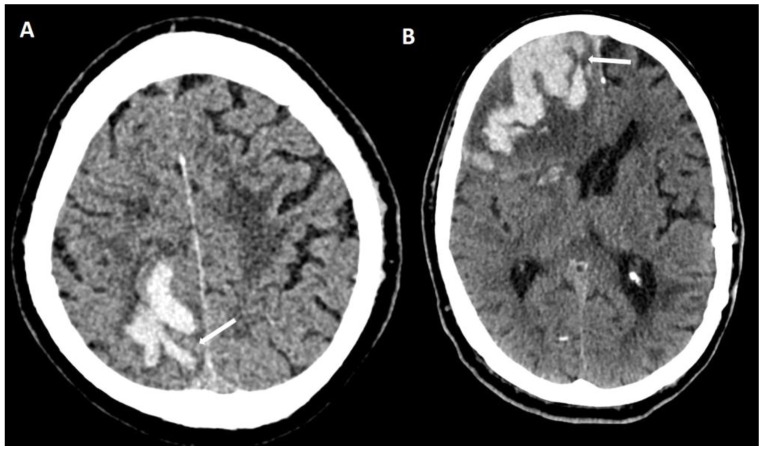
Typical examples of finger-like projections (white arrows) on non-enhanced CT ((A) 100 kV, 394 mAs, slice thickness 1 mm; (B) 120 kV, 320 mAs, slice thickness 1 mm).
